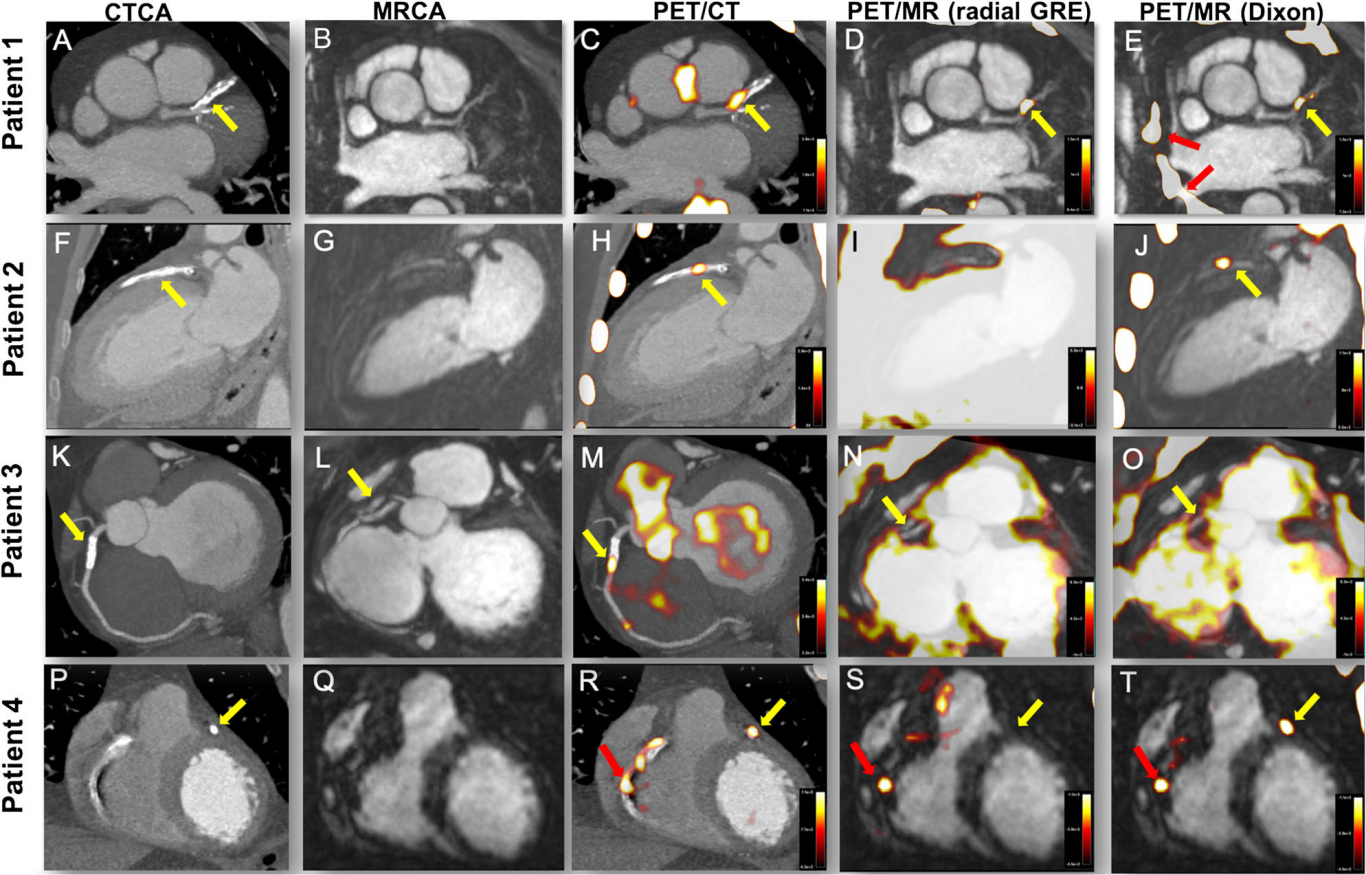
Influence of stents on coronary artery ^18^F-fluoride uptake. Each row represents a patient and the columns the imaging modality. Patient 1: Axial view of complex calcified plaque in the proximal left anterior descending artery (pLAD) (yellow arrow, A) on CCTA. The corresponding CMRA can be appreciated in (B). PET/CT (C) shows focal uptake overlying the complex plaque in the pLAD (yellow arrow) and uptake in the medial wall of the aorta. Both the radial GRE (D) and Dixon PET/MR (E) demonstrate focal uptake within the LAD plaque (yellow arrows) as the PET/CT. However, note the absence of uptake within the aorta demonstrating the utility of later imaging in improving signal-to-noise ratios. Also note airway artifact behind the behind the left atrium and superior vena cava (red arrows) with the Dixon PET/MR (E). Patient 2: Anterior myocardial infarction with primary PCI to the LAD. (F) The 2-chamber view of the metallic stent on the CCTA (yellow arrow). (G) The corresponding CMRA. Focal ^18^F-fluoride uptake can be appreciated on the PET/CT within the body of the stent (H, yellow arrow). (I) The radial GRE PET/MR affected by severe PET dropout over the whole stent despite marked amplification of the blood pool. Employing the Dixon AC map sees the culprit artery signal within the LAD stent return (J, yellow arrow). Patient 3: Modified short-axis CCTA with stent in the proximal RCA (yellow arrow). (L) The corresponding CMRA image of the stent (yellow arrow). (M) Focal ^18^F-fluoride uptake over the body of the stent (yellow arrow) on PET/CT. Radial GRE PET/MR shows PET dropout over the body of the stent (N, yellow arrow). Similarly, Dixon PET/MR is affected by the same artifact precluding assessment of PET activity within the stent (O, yellow arrow). Patient 4: Short-axis CCTA (P) shows a diseased right coronary artery (RCA) and a stent placed in the pLAD (yellow arrow) after primary PCI. Corresponding CMRA can be appreciated in (Q). Uptake within the proximal to mid RCA (red arrow) and culprit LAD (yellow arrow) can be appreciated on the PET/CT (R). PET/MR also shows focal ^18^F-fluoride uptake in the mid RCA with radial GRE PET/MR (S, red arrow) but note the absence of LAD uptake in the region of the stent (yellow arrow). On Dixon PET/MR (T), the LAD uptake is visible (yellow arrow) alongside focal RCA uptake (red arrow)
